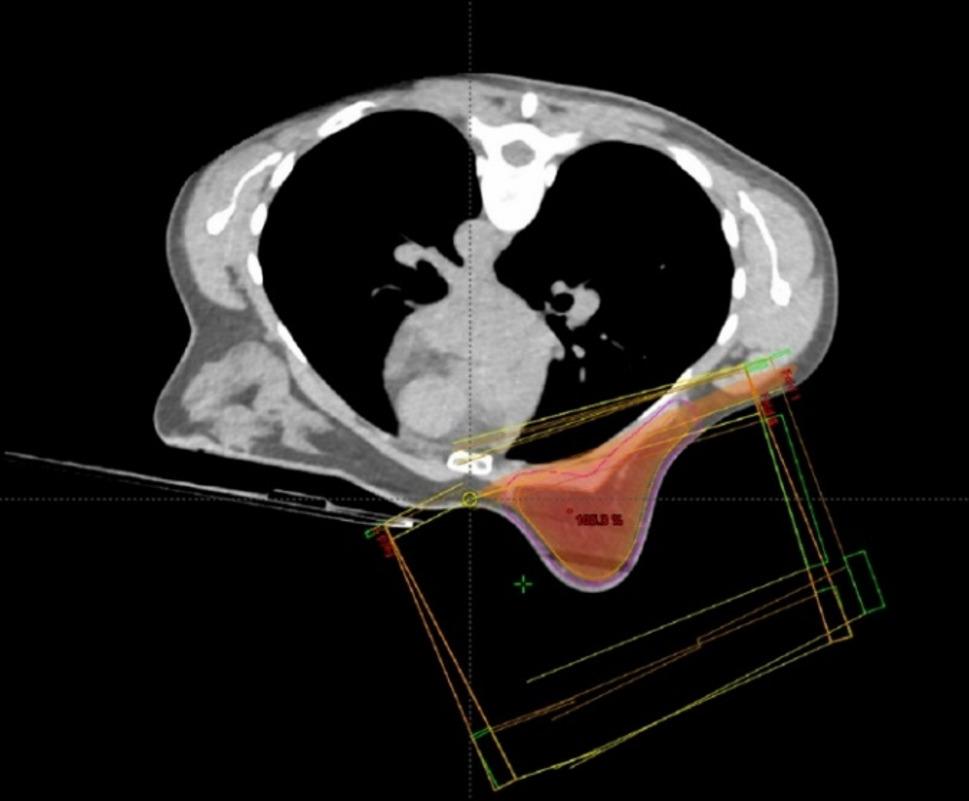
CT-scan from the investigated cohort with a smaller breast size in the prone position. Note: The orange line indicates clinical target volumes (CTV), and the red line indicates planning target volumes (PTV). The orange area represents the applied dose of the radiation plan. White lines indicate irradiation-fields
